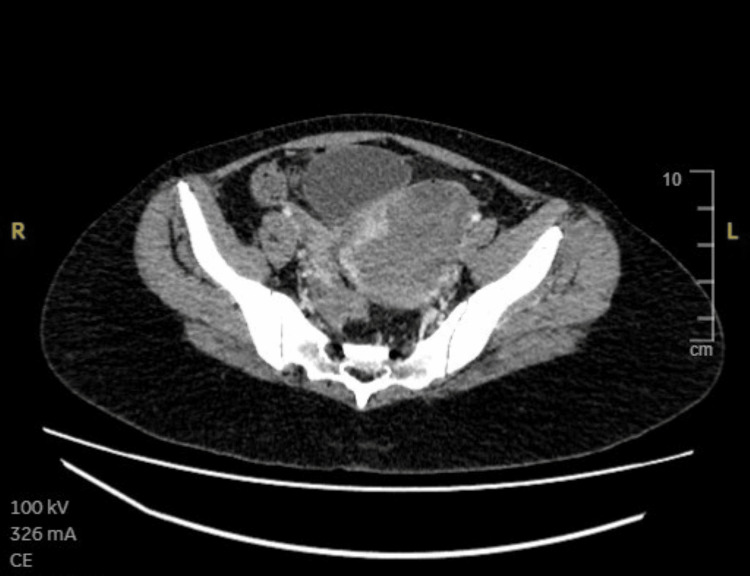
CT scan reported as post-miscarriage for twins. There is an intrauterine collection, containing heterogeneous material in keeping with intraluminal residual hemorrhage.
